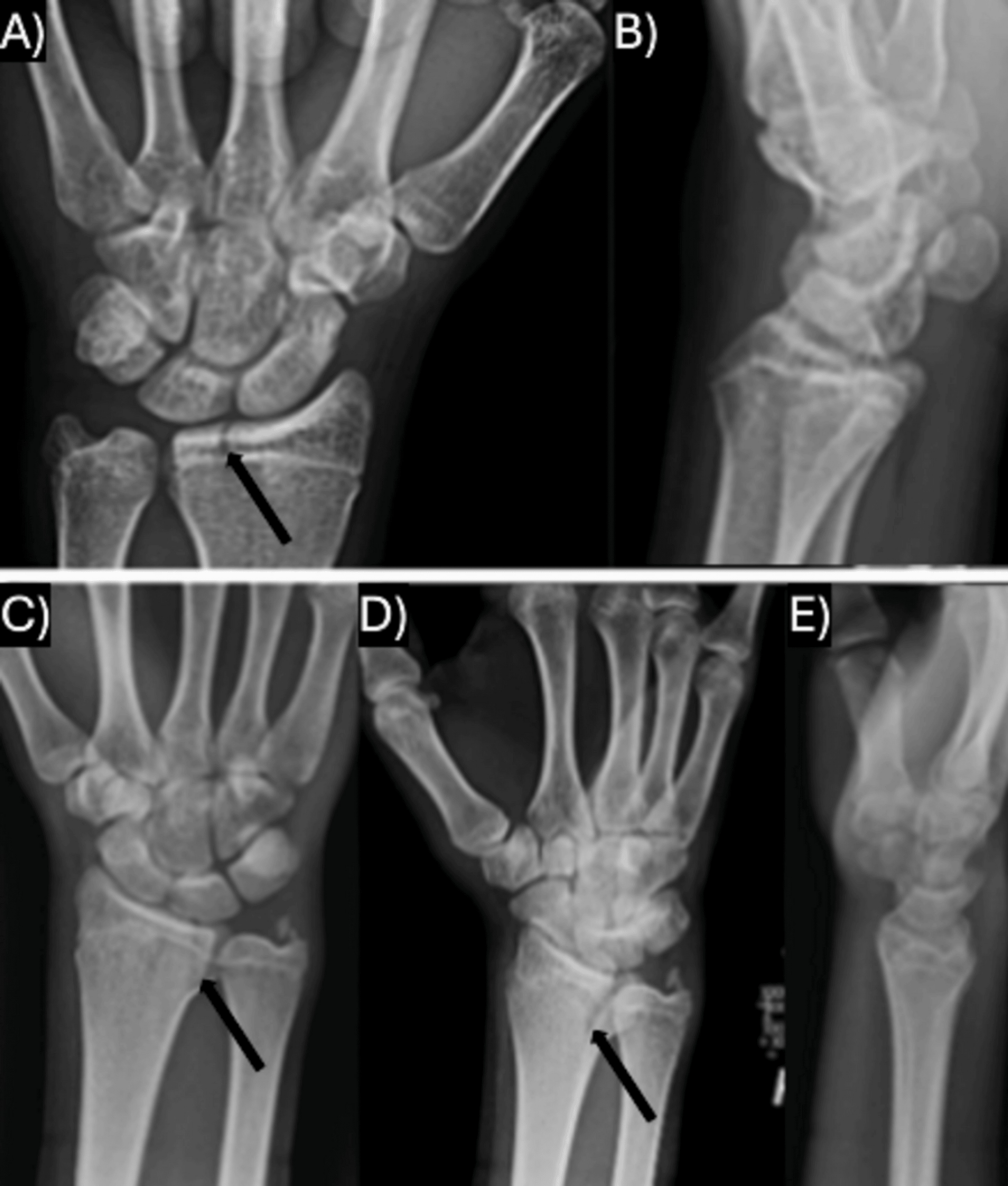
Injury films of cases with isolated SH-III components on AP radiographs and no fracture lines on lateral radiographs A) Case III's AP radiographs; B) Case III's lateral radiographs demonstrating no fracture line. C) Case V's AP radiographs; D) Case V's oblique radiographs; E) Case V's lateral radiographs demonstrating no fracture line. For Figures A, C, and D, the black arrow indicates the isolated SH-III component on the AP/oblique radiographs. SH: Salter-Harris; AP: anterior-posterior
